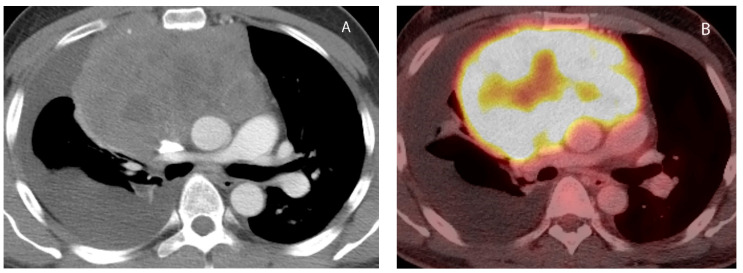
Primary mediastinal lymphoma. CT (A) shows large heterogeneous soft tissue mass in the prevascular mediastinum. The mass is hypermetabolic on FDG PET-CT (B). Biopsy showed diffuse large B cell lymphoma.
